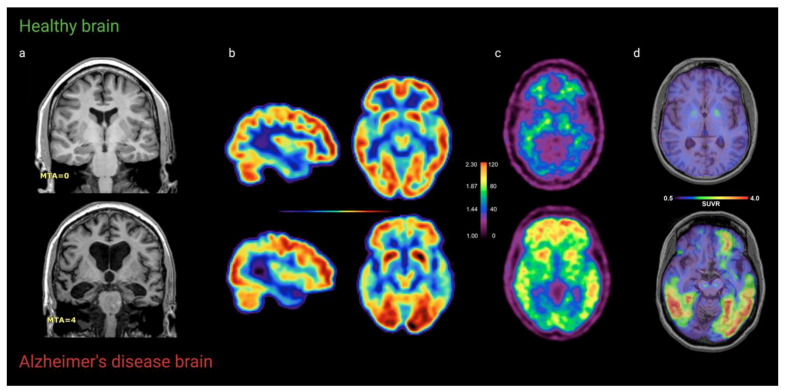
Neuroimages of the healthy versus the Alzheimer’s disease (AD) brain. Neuroimaging with (a) structural MRI, (b) FDG-PET, (c) amyloid-PET with PiB and (d) tau PET with ^18^F-AV1451 in both healthy and AD brains. Figure created with www.BioRender.com (accessed on 14 February 2021).
